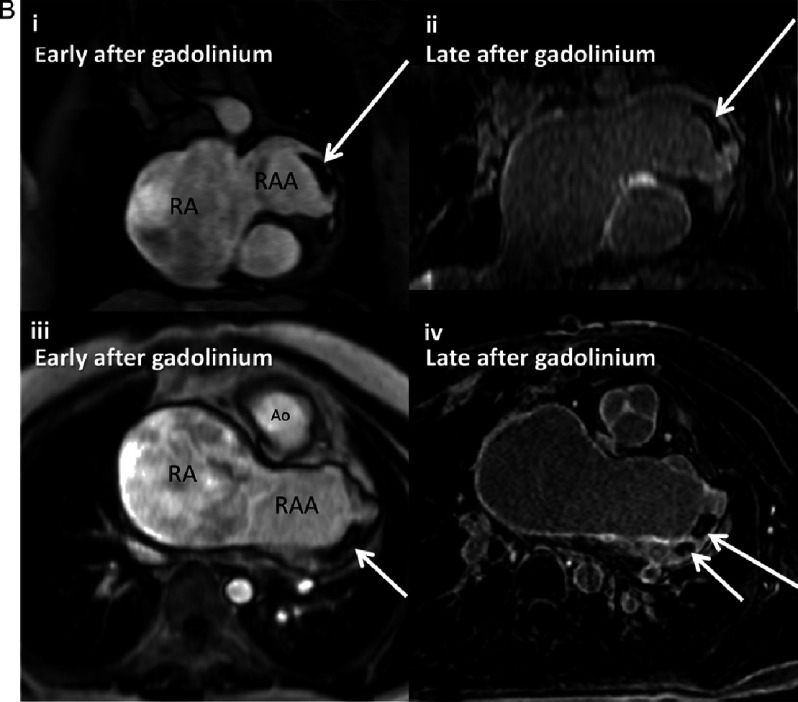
(A) Patient 1 imaging findings. (i) Chest radiography in posteroanterior view, showing bulging (white arrows) of left heart contour below the left pulmonary artery, as a result of left juxtaposition of the atrial appendages. Situs solitus is inferred from the normal bronchial anatomy and cardiomegaly is noted. (ii) Corresponding coronal image from 3D balanced steady state free precession (3D bSSFP). Grossly dilated right atrium and enlarged, left juxtaposed right atrial appendage (white arrows) characterised by pectinate muscles are noted. The darker spot amongst the pectinate muscles is thrombus - see Figure 1B. (iii) Right atrial dilatation with sluggish blood flow on still frame from cine CMR, axial view, also demonstrating the underlying tricuspid atresia. (iv) 3D bSSFP sagittal image showing dilated right atrial appendage (RAA) in the left hemithorax. Underlying transposition of great arteries can be noted with anterior aorta from the RV. CMR, cardiovascular magnetic resonance; RA, right atrium; RAA, right atrial appendage; Ao, aorta; PA, pulmonary artery; LPA, left pulmonary artery; RV, right ventricle; LV, left ventricle; LA, left atrium. (B) Contrast-enhanced CMR findings documenting thrombus in patient 1. Early after gadolinium injection, coronal (i) and axial views (iii) show dilated right atrium and appendage and a filling defect (dark region, white arrow) at the left tip of the right atrial appendage which is typical of thrombus. Corresponding coronal (ii) and axial (iv) image planes confirm low signal (darker) in the same region again consistent with thrombus within the right atrial appendage, (white arrows). CMR, cardiovascular magnetic resonance; RA, right atrium; RAA, right atrial appendage; Ao, aorta.
